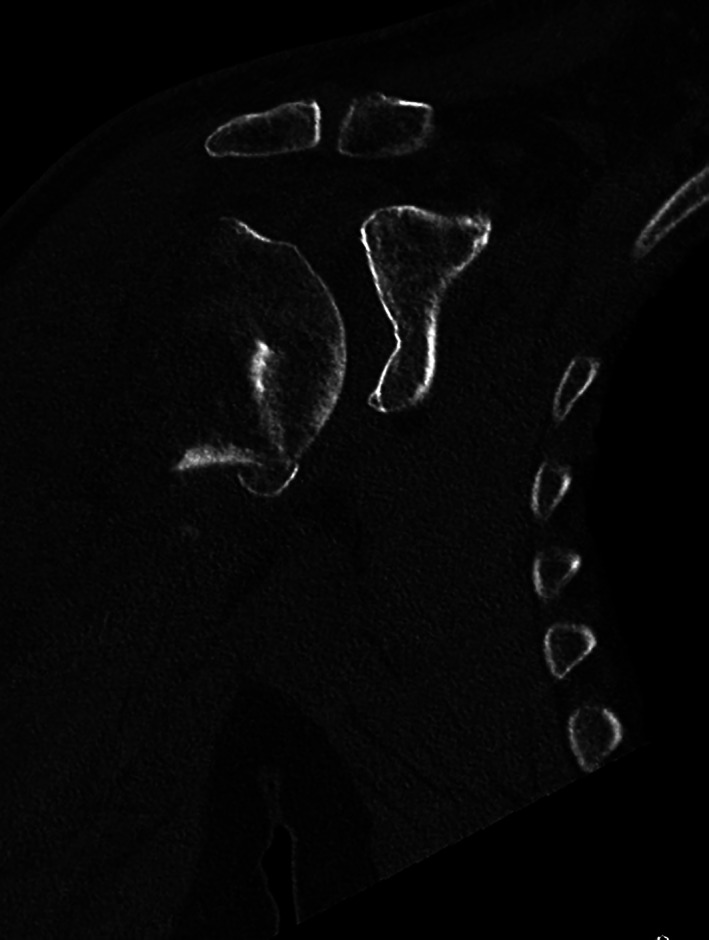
CT image depicting a glenoid dent often associated with dysplastic glenoids.
